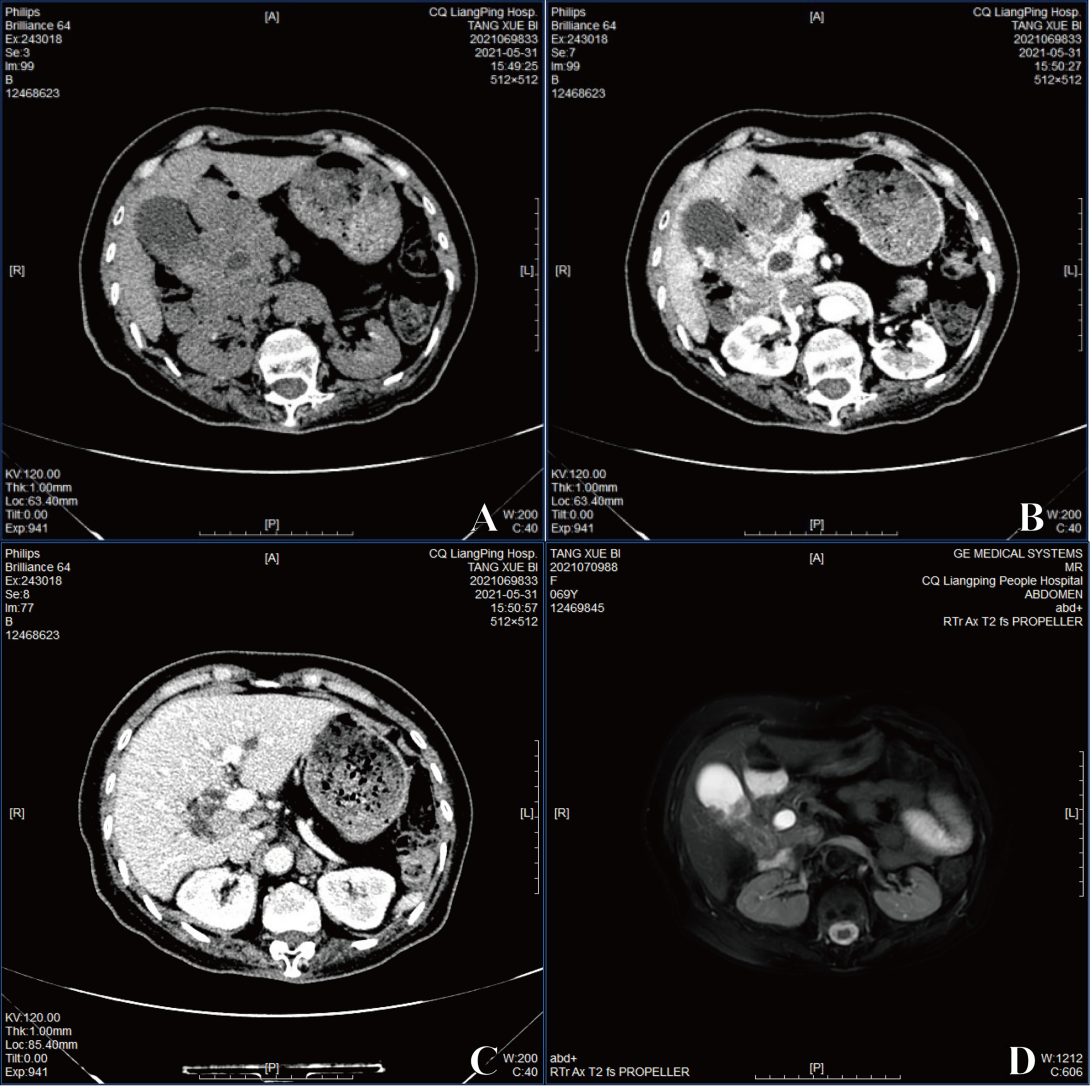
(A) The image exhibited with CT. (B) The image of enhanced CT. (C) Porta hepatis lymph node enlargement by enhanced CT. (D) The image of MRI.
